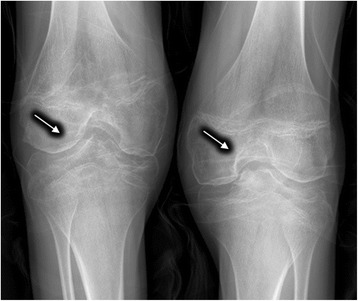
The knee plain film of knee joints showed degenerative changes with articular surface hyperosteogeny and swelling joint capsule (*Arrows*)
